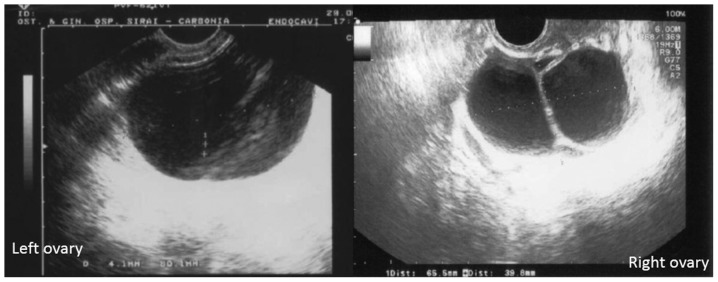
Case 1: Bilateral ovarian cysts. Transvaginal ultrasonography examination at admission showed a right multilocular ovarian cyst (85×40 mm) and a left multiloculated mass (65×46 mm).
